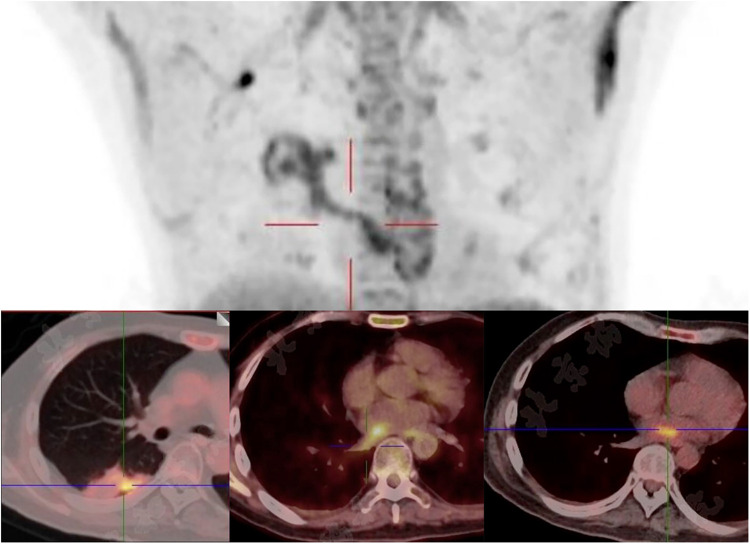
Focal infectious uptake was detected in the right lower lobe dorsal segment on PET/CT. The SUVmax is 5.0 in the pulmonary lesion, 5.3 in the left atiral lesion, and 4.9 in the RIPV.
